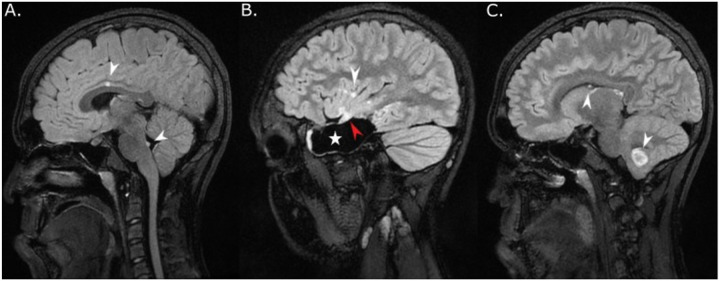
MRI of patient 9 with multifocal DNET and leptomeningeal dissemination. (A) Sagittal FLAIR post contrast image showed enlarging enhancing nodules in the dorsal brainstem (lower white arrowhead) and in the inter-hemispheric fissure (upper white arrowhead) compatible with progressive leptomeningeal disease 7 years after diagnosis of multifocal dysembryoplastic neuroepithelial tumor (WHO grade 1) with primary tumor in the right temporal lobe. (B) Sagittal FLAIR post-contrast image obtained on the same day showed residual hyperintense right temporal lobe tumor (red arrowhead) and surgical cavity (white star). Additional enhancing leptomeningeal punctate lesion was also seen in the right frontal lobe (white arrowhead). (C) Sagittal FLAIR post contrast image obtained on the same day showed a second distinct lesion in the right cerebellum with a typical ring sign (lower white arrowhead) and intra-ventricular punctate enhancing lesion compatible with leptomeningeal spread (upper white arrowhead).
